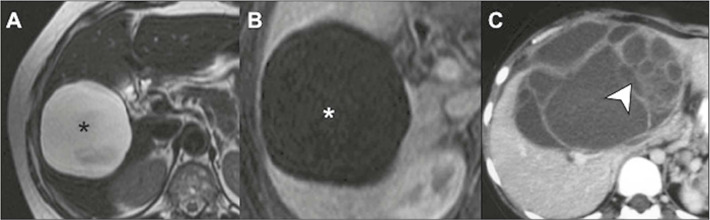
Types I and II hydatid cysts. On MRI, type I (A,B)
